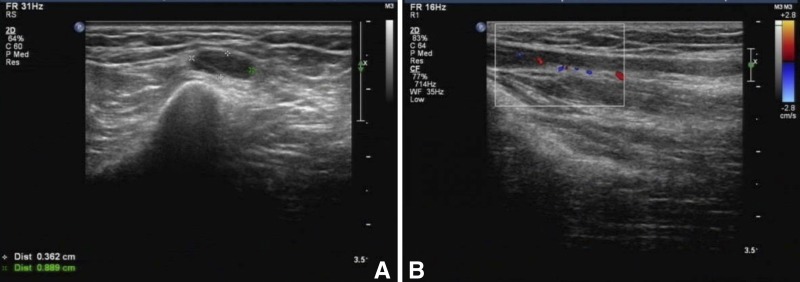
Post-therapy (2 weeks after initiation of multidrug therapy [MDT]) ultrasonography and color Doppler images of peripheral nerve of the patient. (A) Cross-section scan of the right side ulnar nerve with hypoechoic fascicles: 0.889 cm at its widest point and (B) abnormal blood flow signals around the right ulnar nerve.
